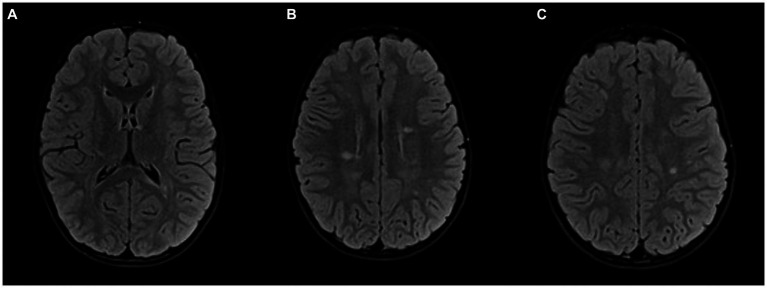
Follow-up MRI after 2 years: FLAIR sequence in axial plane at the same levels as in Figure 2
